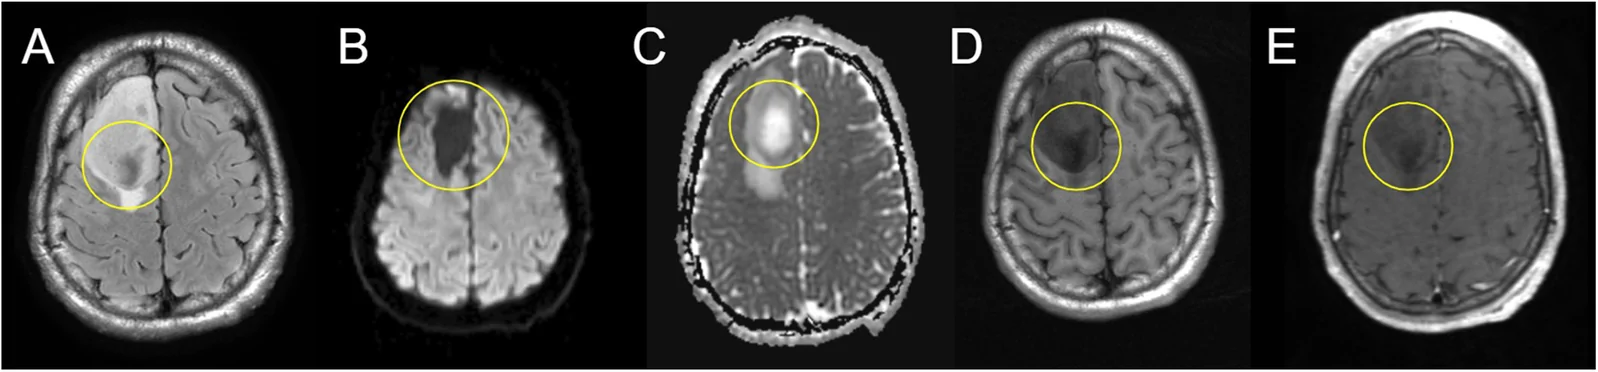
Low-grade glioma. (A) Axial FLAIR demonstrates a hyperintense intraaxial lesion within the high right anterior frontal lobe (yellow arrow). (B) Axial DWI reveals no restriction (yellow arrow). (C) ADC map shows higher value of the lesion (yellow arrow). (D) Axial T1-weighted pre-contrast MRI shows hypointense lesion (yellow arrow). (E) Axial T1-weighted post-contrast MRI shows no abnormal enhancement (yellow arrow).
